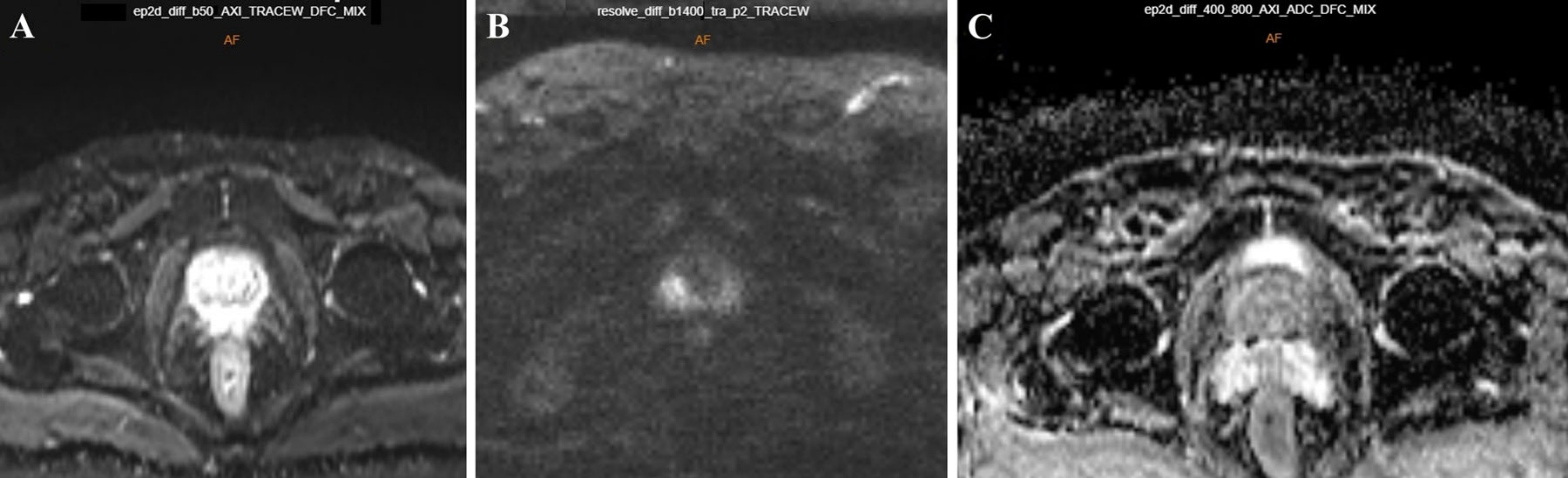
DWI acquired at b‐value 50 s/mm^2^ (A), *b*‐value 1400 s/mm^2^ (B) with ADC map (C). High *b*‐values result in the suppression of the background normal prostate signal, enabling the visualization of cancerous lesions as bright areas.
